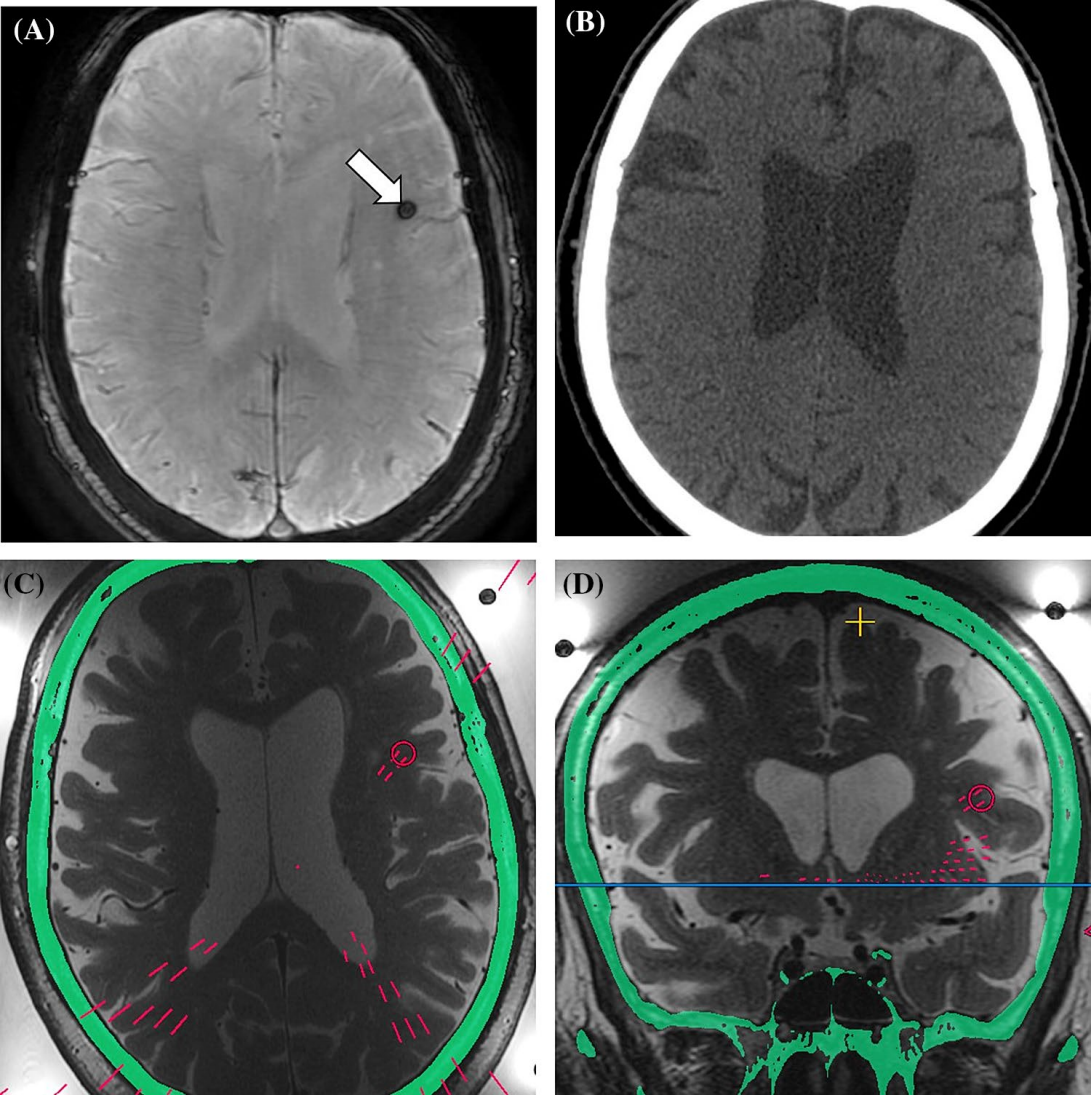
70-year-old man with essential tremor. Preoperative SWAN sequence detects a hypointense lesion in the left frontal lobe (A, arrow), not corresponding to calcification on CT image (B). Axial and coronal intraoperative T2-weighted FRFSE show the no-pass region that was placed during treatment planning [red circles in (C) and (D)], blocking 4 additional elements (4.6% of the total elements turned off).
